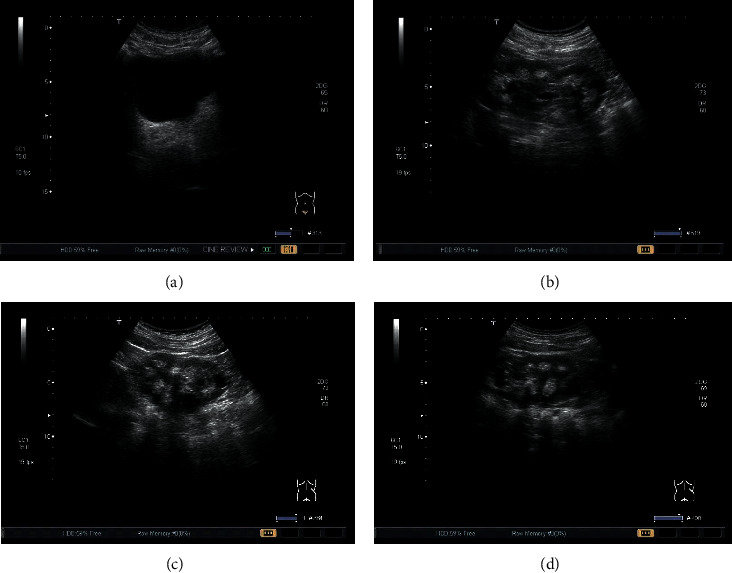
Ultrasound images based on artificial intelligence PCNN algorithm. (a) Ultrasound image of renal cortex thinning and echo enhancement. (b) Ultrasound image of renal cortex and medulla echo being unclear. (c) Cortical echo enhancement and an ultrasound image of renal enlargement. (d) Ultrasound image of renal inferior pole laceration and subcapsular effusion.
